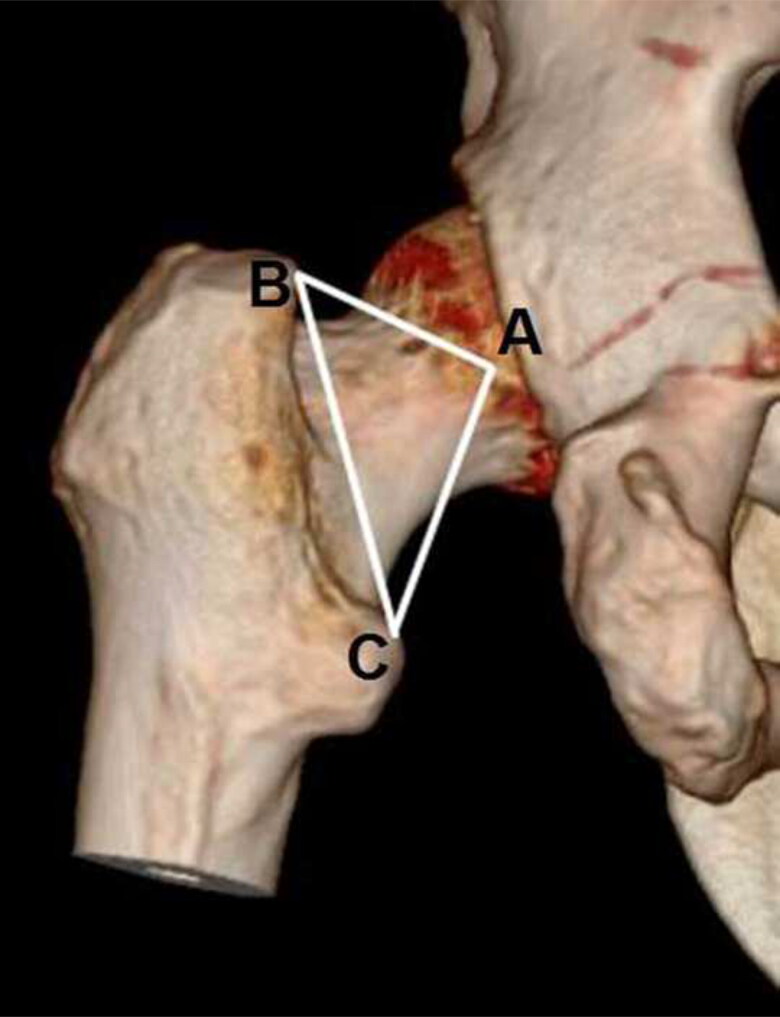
Purkait’s triangle landmarks taken from a CT-scans sample. Point A: The most lateral projecting landmark of the articular facet on the femoral head, Point B: Corresponds to the most medially projecting point of the greater trochanter, and Point C: The most posteromedial point of lesser trochanter.
